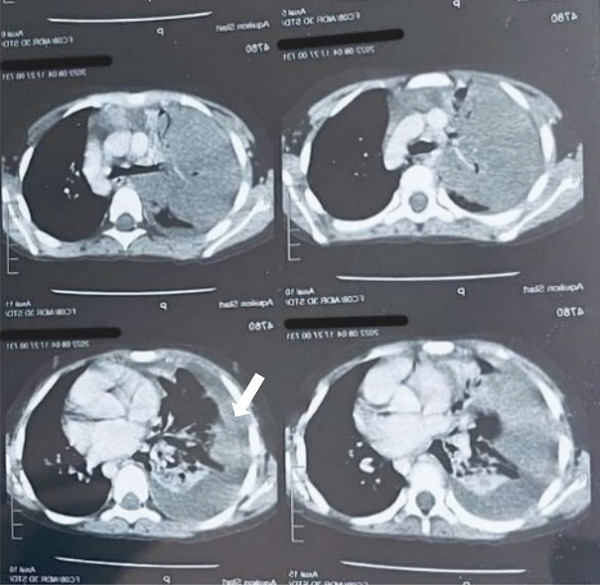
CT chest sagittal plane showing right‐sided empyema (white arrow).
